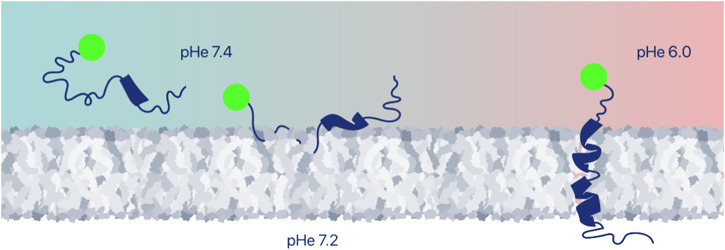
Extracellular delivery of imaging or therapeutic payloads. Various payloads can be positioned at cell surfaces within tumors by conjugation to the pHLIP terminus that remains outside of the cell. Among the useful payloads are fluorescent dyes, PET and SPECT imaging agents, various antigens, or immune-cell-recruiting molecules, and proteins. Peptide and protein payloads might be expressed with pHLIP as a single polypeptide.
